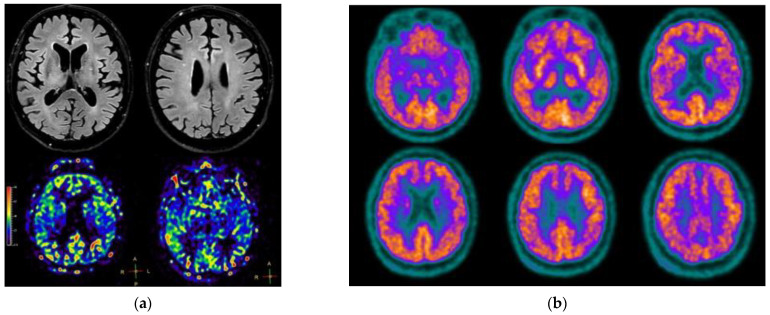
Imaging findings at last follow up: (a) Brain MRI—upper panels showing axial FLAIR slices, lower panel showing normal brain perfusion on axial arterial spin labeling slices; (b) FDG-PET.
